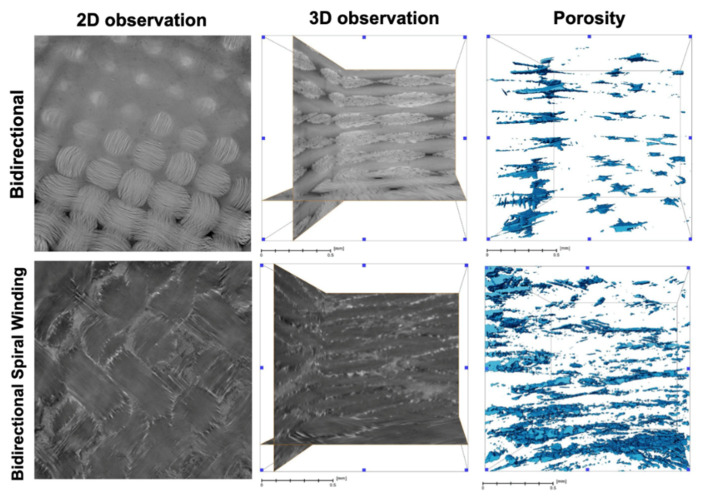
Volume rendering of the segmented pores (blue color) in bFRC and bswFRC, obtained by X-ray tomography analysis. The scale bar corresponds to 0.5 mm in all images.
